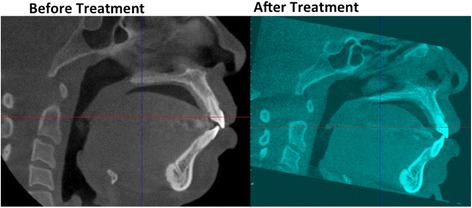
Pretreatment (*black* and *white*) and posttreatment (*blue*) CBCT images of a high-angle patient. Note that the central incisor has been pushed past the buccal cortical plate after orthodontic treatment
